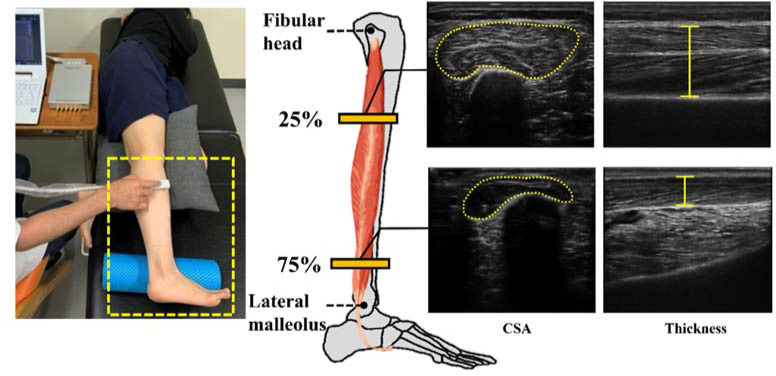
Peroneus muscles’ CSA, thickness, and echogenicity of the proximal 25% (indicates the morphology of the PL) and distal 75% (indicates the morphology of the PB) in a straight line connecting the fibular head and the lateral malleolus were measured using an ultrasound imaging system. PL: peroneus longus, PB: peroneus brevis, CSA: cross-sectional area
